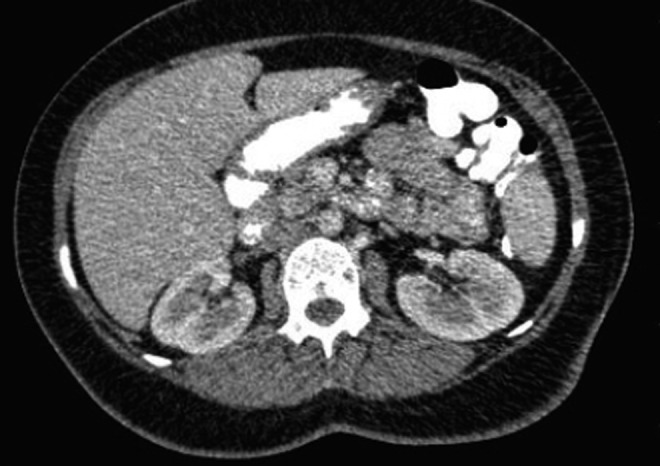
Postoperative CT scan of the patient.
